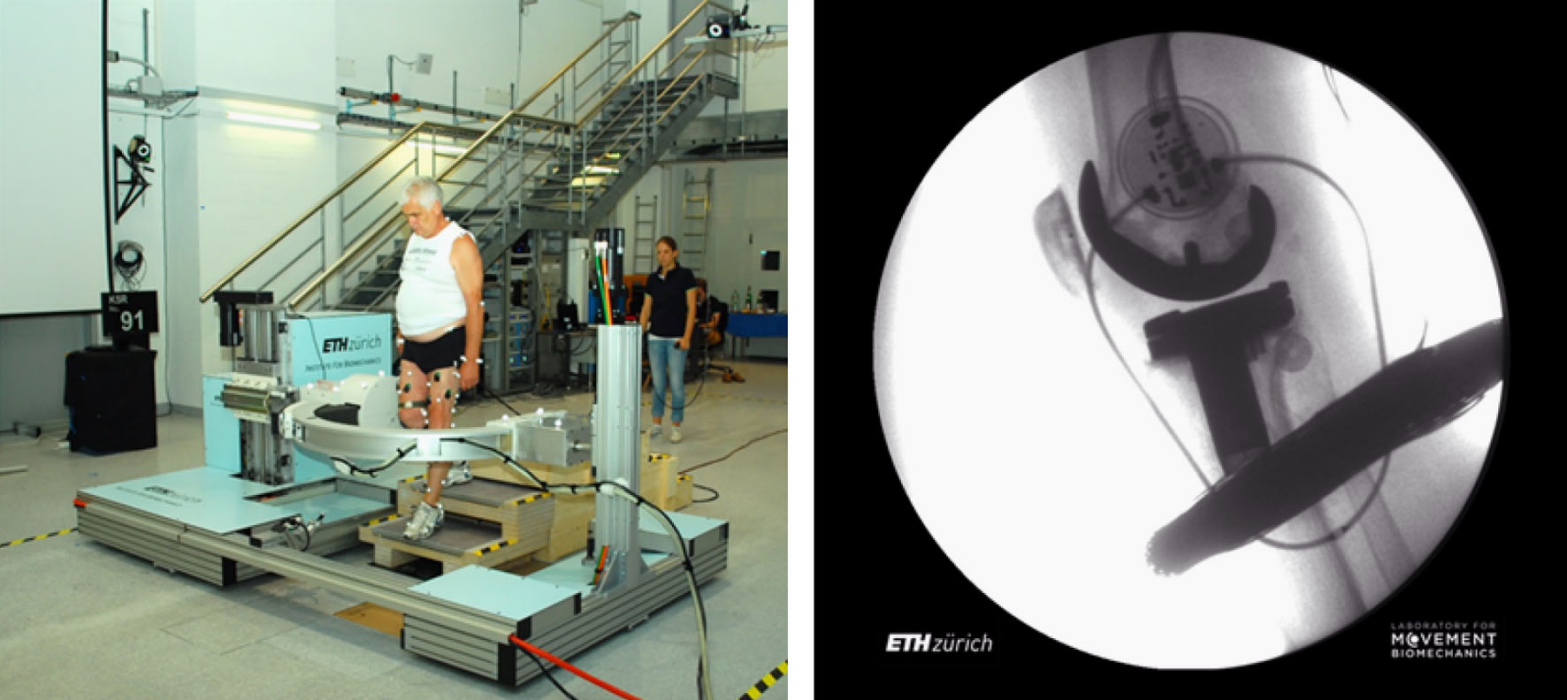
The ETH moving fluoroscope was used to capture radiographic images of the knee joints throughout a range of dynamic activities (left). An exemplary fluoroscopic image including the implant component projections is shown on the right. Specific informed consents were acquired from the subjects in order to publish their images.
